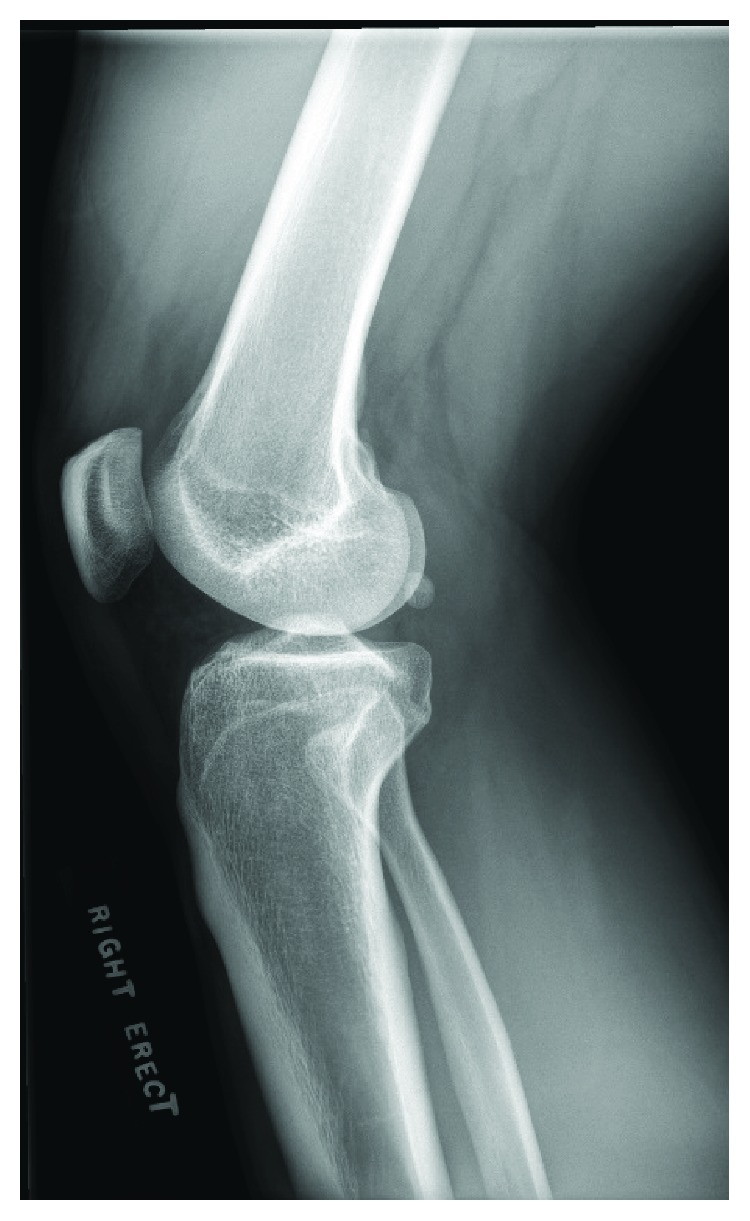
X-ray showing the fabella in a normal posterior location on the lateral X-ray.
